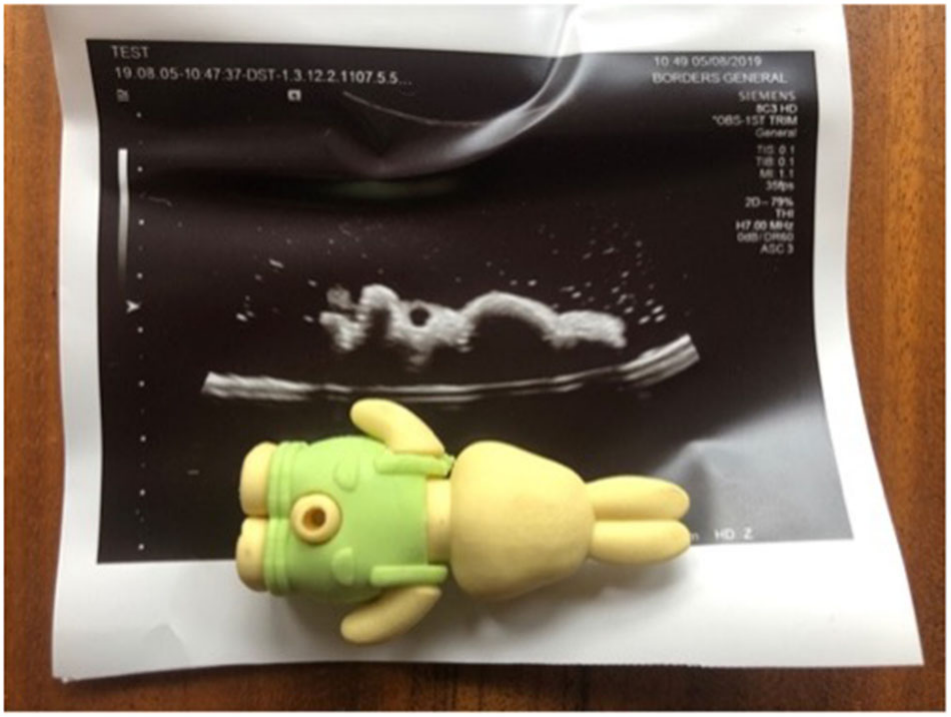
Example of Rubber Eraser and Its Appearance on Ultrasound
